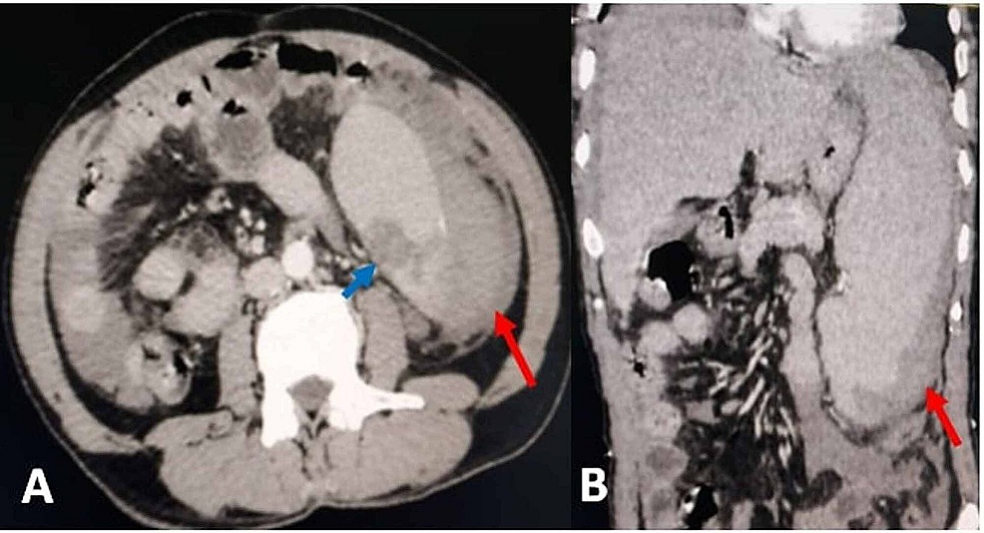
CECT showing a grade three splenic laceration 3.3 × 2.6 cm in the lower pole (blue arrow) and subcapsular hematoma involving >50% of the surface area (red arrow) with hemoperitoneum. A: axial section; B: coronal section CECT: contrast-enhanced computed tomography
